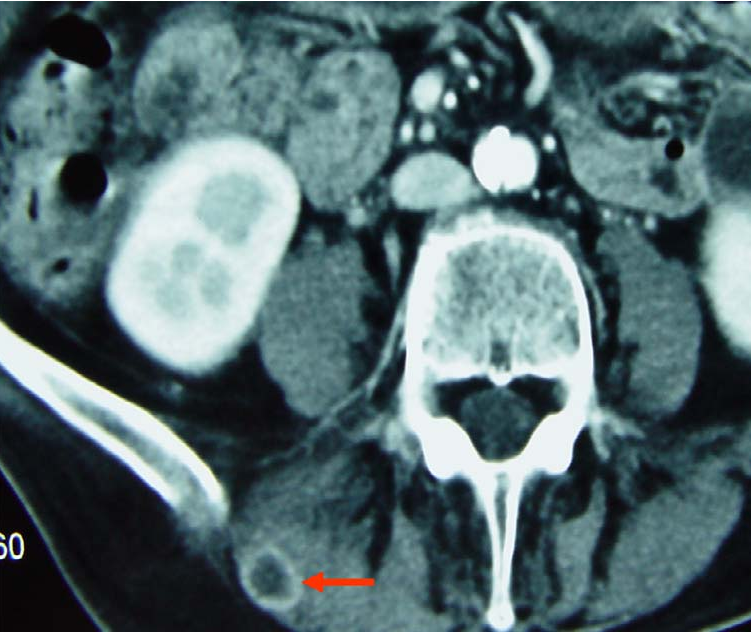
Contrast enhanced CT, soft tissue window: Soft tissue metastasis (1 cm) in the iliocostal lumborum muscle on the right side.
